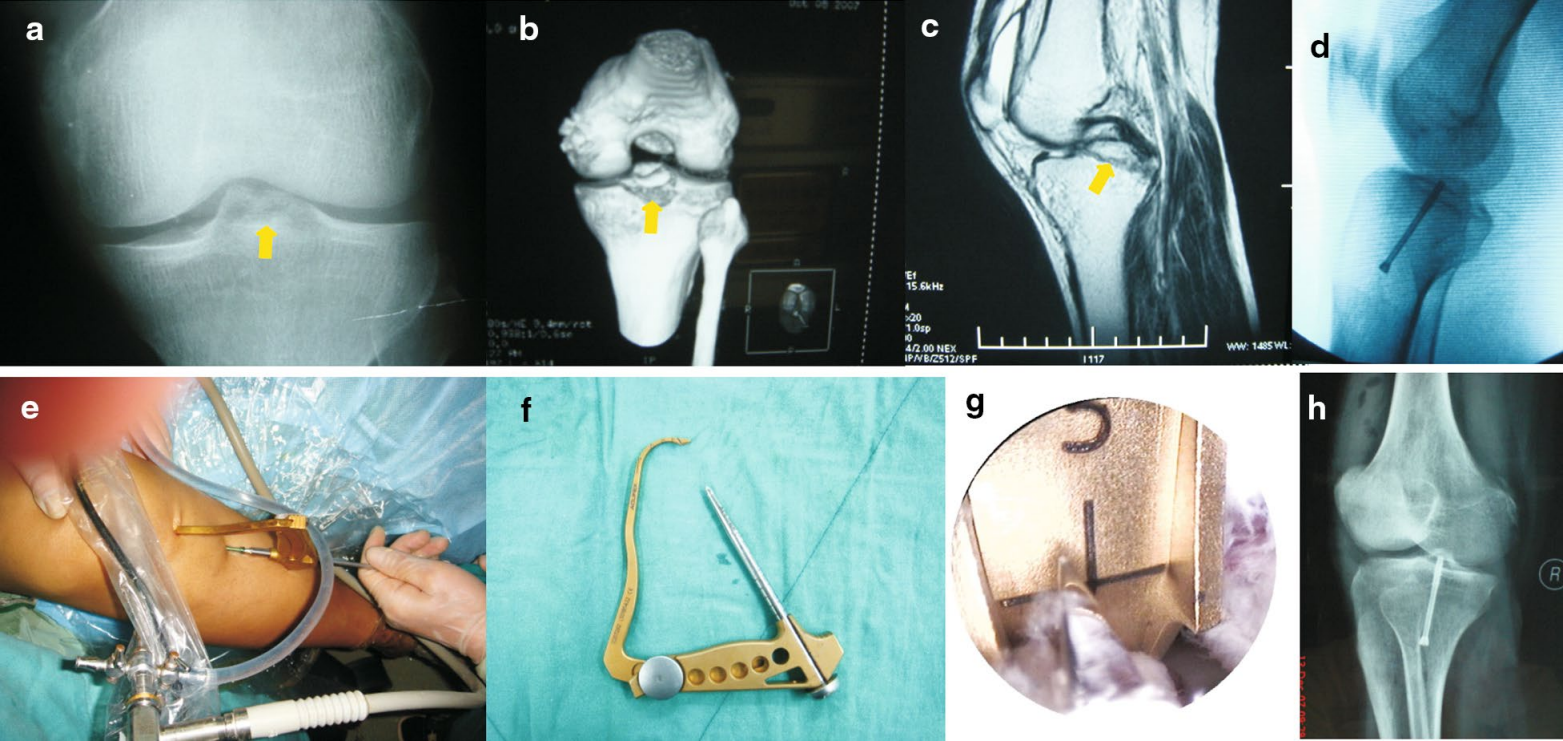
PCL avulsion fracture of a 26-year-old male was fixed anteriorly under arthroscopy with one partially threaded cannulated screws. a, b X-ray and CT showed the tibial intercondylar eminence fracture. c MRI showed the tibial intercondylar eminence fracture of PCL insertion. e–g The tibial PCL guide (Acufex) was used to secure the fracture fragment and guide one kirschner wire. d, h The position of one partially threaded cannulated screw was confirmed under image intensifier and X-ray examination after operation. *Arrow* pointed the fracture
